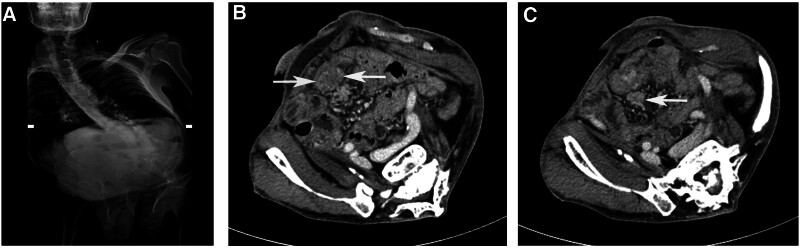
Images of spinal X-ray and abdominal CT. (A) Spinal X-ray indicates severe scoliosis. (B) Abdominal contrast-enhanced CT shows slight thickening and strengthening of the small intestine wall in the right low quadrant (arrow). (C) Abdominal CT shows a mesenteric mass near the primary disease (arrow).
